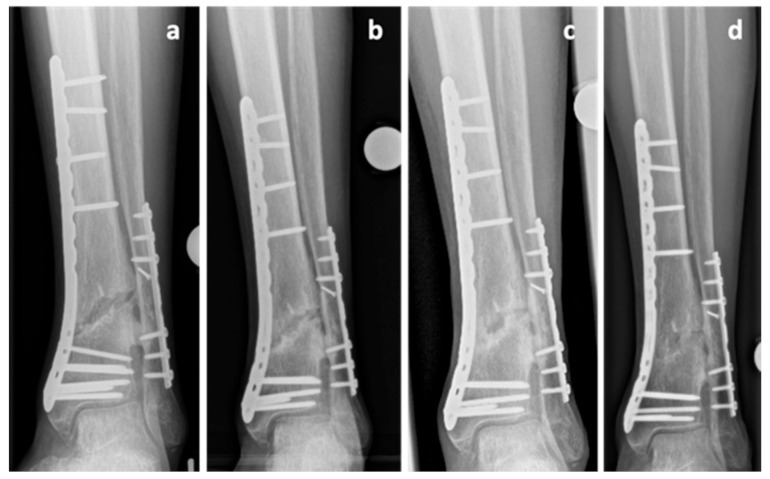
Case 4, x-rays showing a nonunion 29 months after the initial injury at the initiation of the teriparatide therapy (a). Serial x-rays after 3 and 15 months of teriparatide treatment showing gradual callus build-up (b,c). Radiological and clinical union was achieved 4 years after the initial injury (d).
